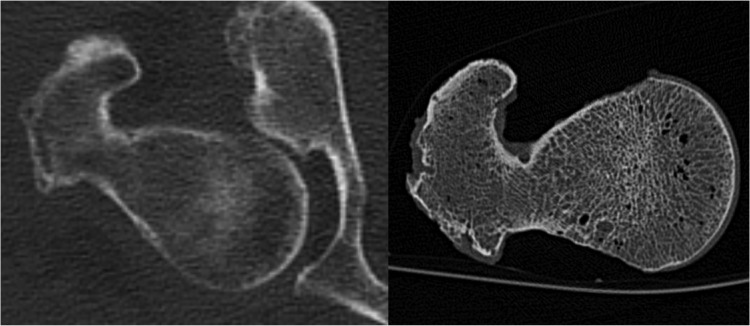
In vivo CT Image of a proximal femur with image noise and artifacts caused by surrounding soft tissue compared to an in vitro CT Image with better depiction of the trabecular and cortical structures. The spatial resolution, determined at ρ50 of the modulation-transfer-function, is 230μmx230μm *in vitro* and 250μmx250μm *in vivo*.
